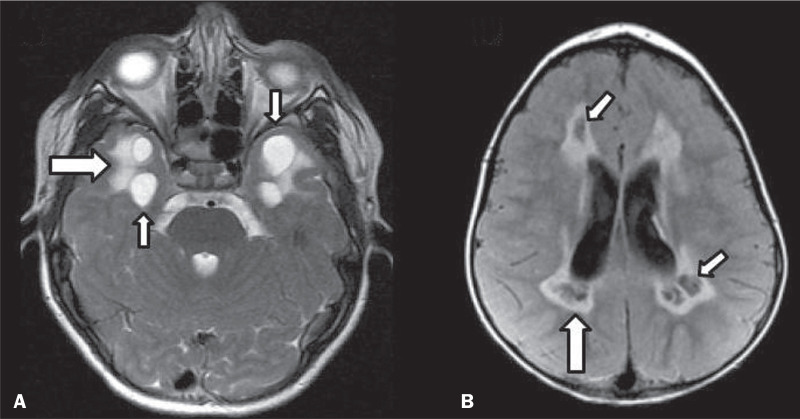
Presence of cysts in INMDs. MRI of the skull. A: T2-weighted axial TSE
